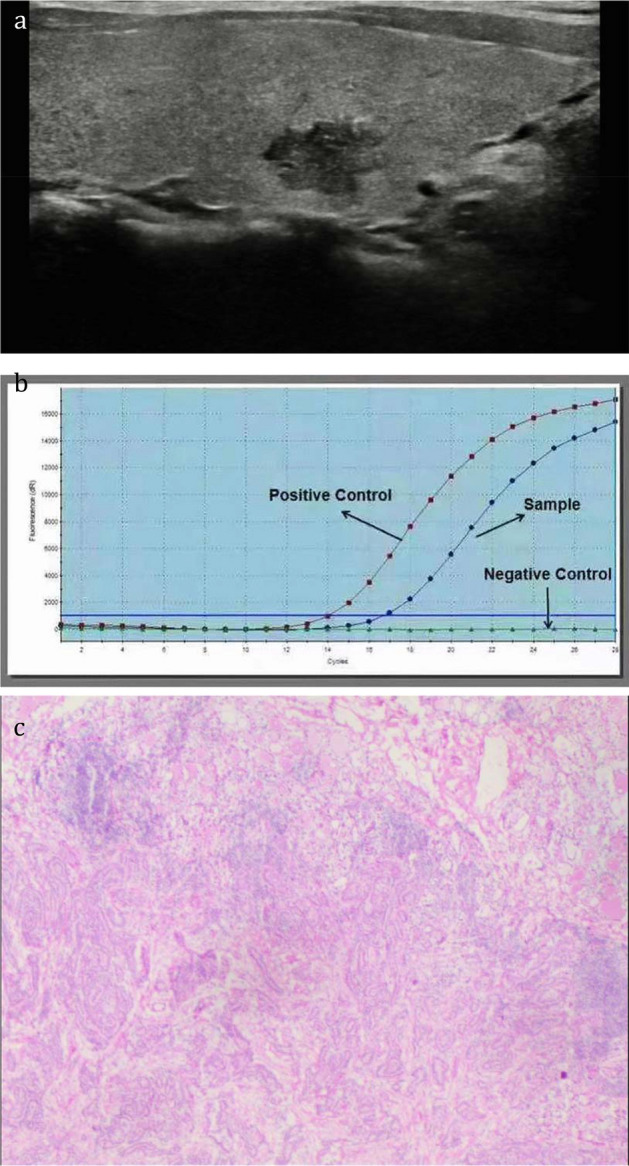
A thyroid nodule, assigned to Bethesda category III, was considered malignant diagnosed by the combination of TIRADS and BRAF^V600E^ analysis. The nodule proved to be a malignant tumour confirmed by postoperative pathology. (a), the 2D sonogram classified as TIRADS 4b, (b), mutant BRAF^V600E^ gene, (c), papillary thyroid carcinoma.
